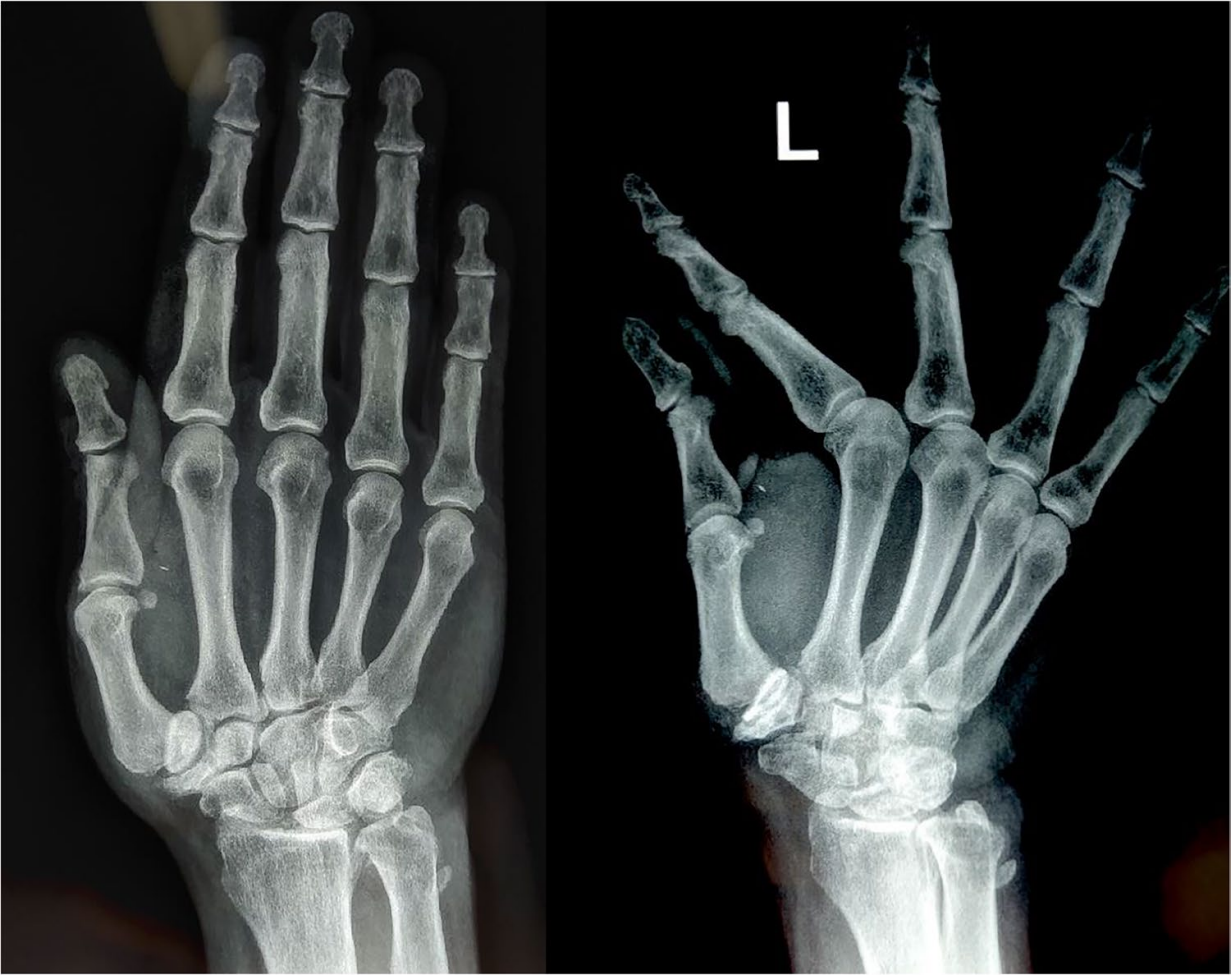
Man, 72 years old, post-operative X-ray at 4-year follow-up with a subluxation of more than 1/4 of the metacarpal base
